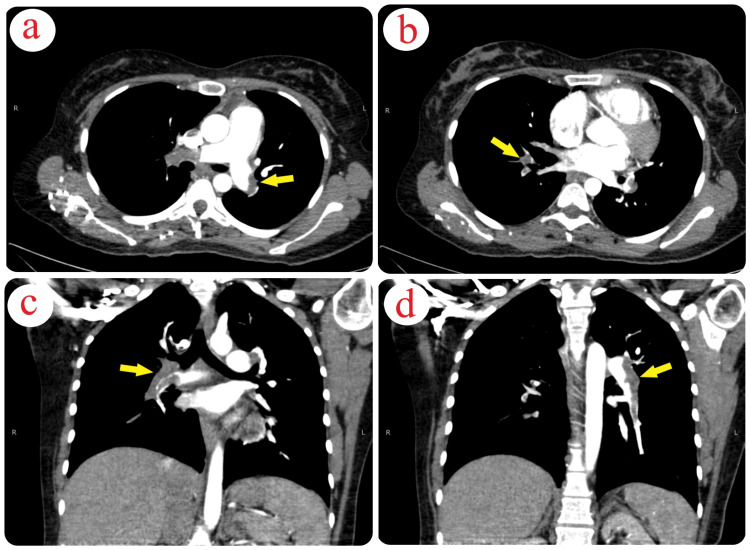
CT Angiogram Indicating Pulmonary Embolism (a-d) The CT angiogram displays filling defects in the pulmonary arteries, consistent with a significant pulmonary embolism.
